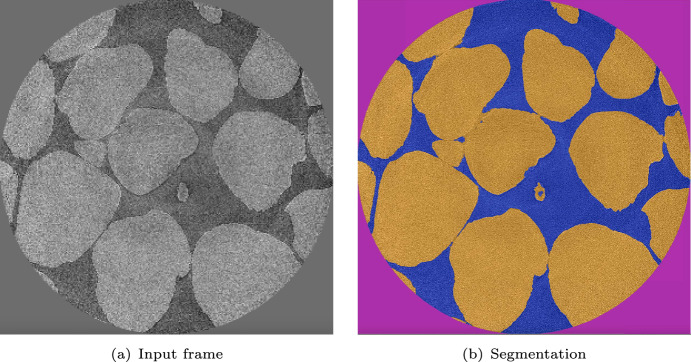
Example of a single frame of the X-ray tomography characterization of sand grains and its segmentation
